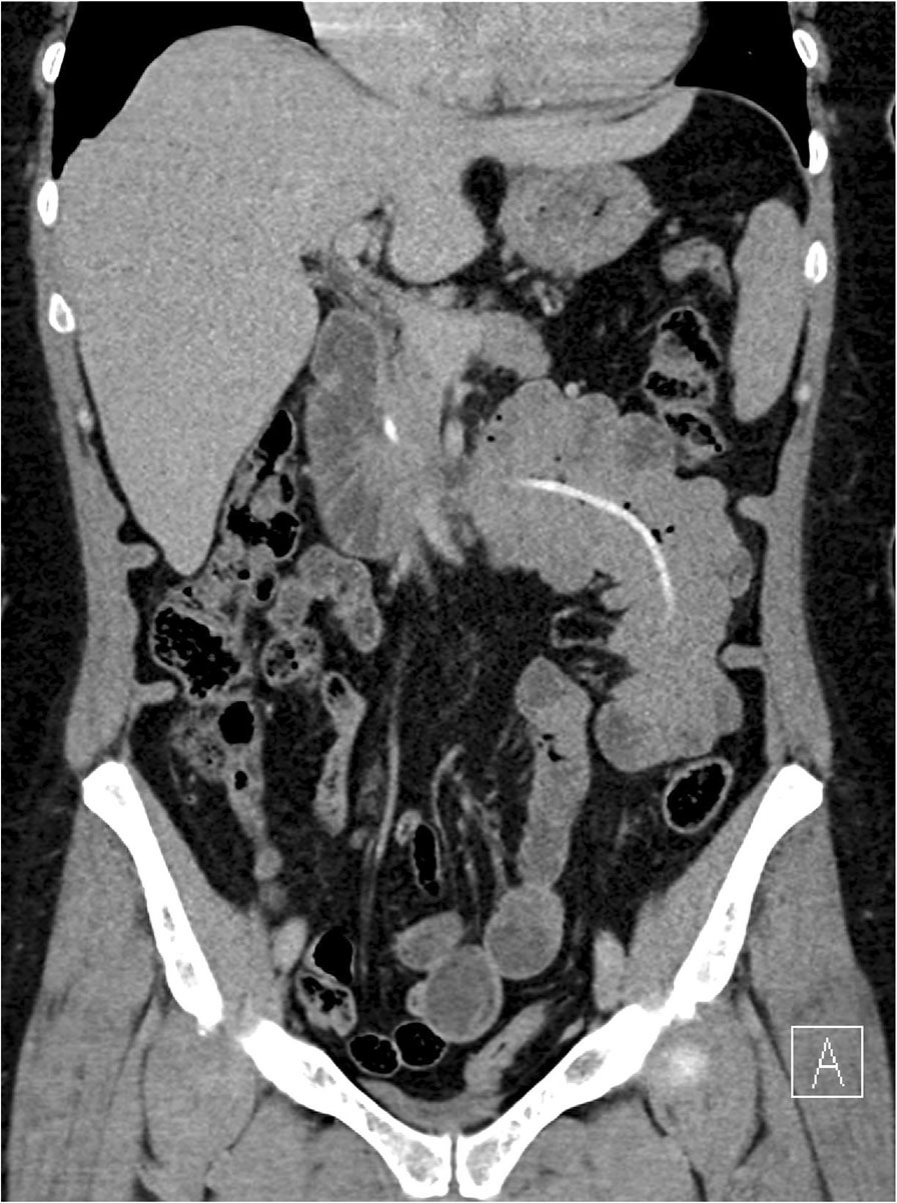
Computed tomography scan with jejunum threaded on the catheter
